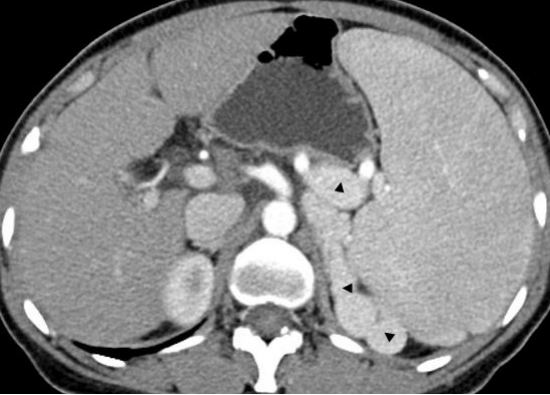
Contrast computed tomography revealing splenomegaly and varicose veins (triangles).
